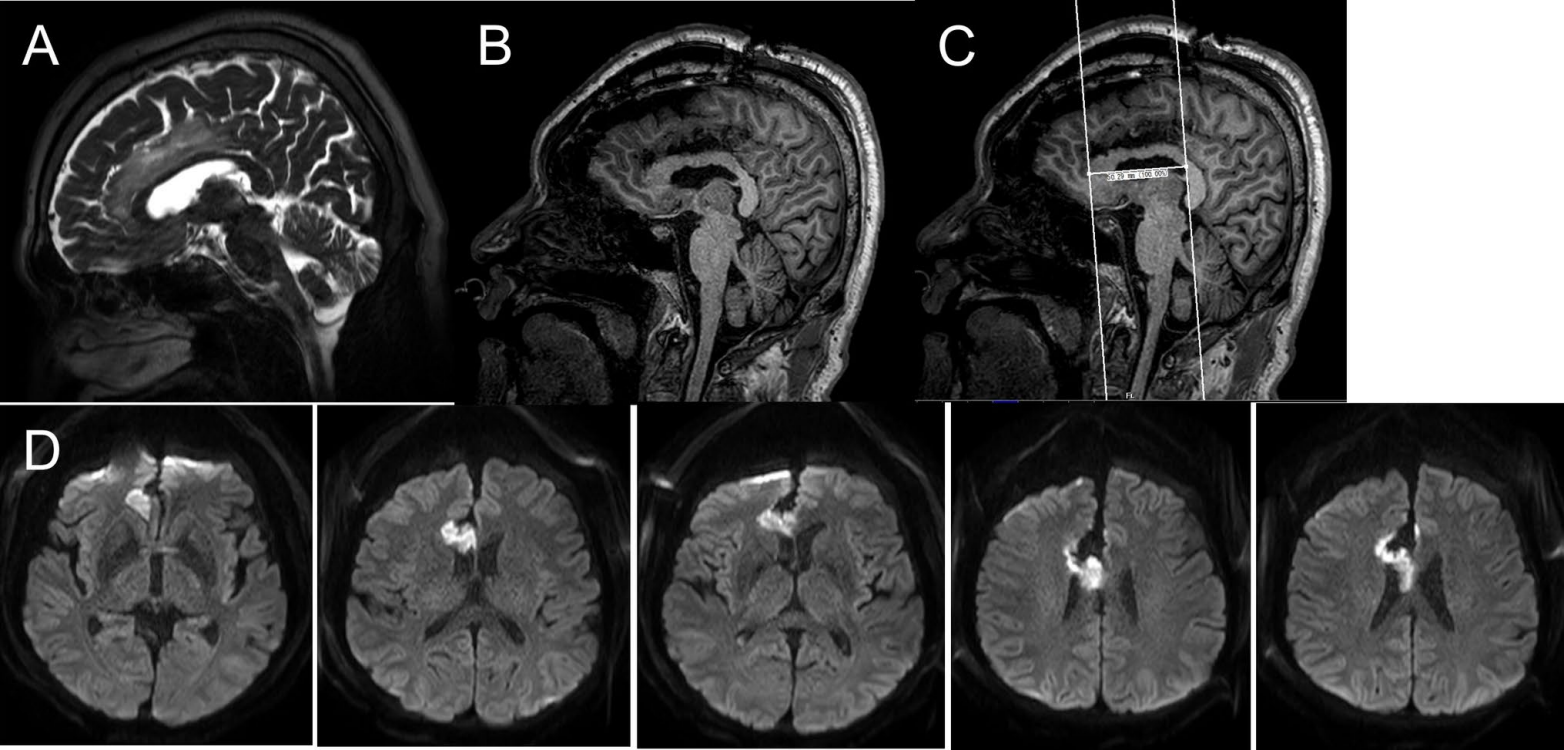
A 67-year-old man underwent an incidental MRI. The cingulate gyrus was enlarged, extended along the long axis, and exhibited a high T2 signal (A). A low-grade glioma was suspected and resection was performed. The entire high T2 signal area was removed (B). The resection extended 5 cm longitudinally (C). Postoperative MRI showed an ischemic area in the corpus callosum lateral to the resected region (D). Postoperatively, he exhibited consciousness disturbances for 7 days
